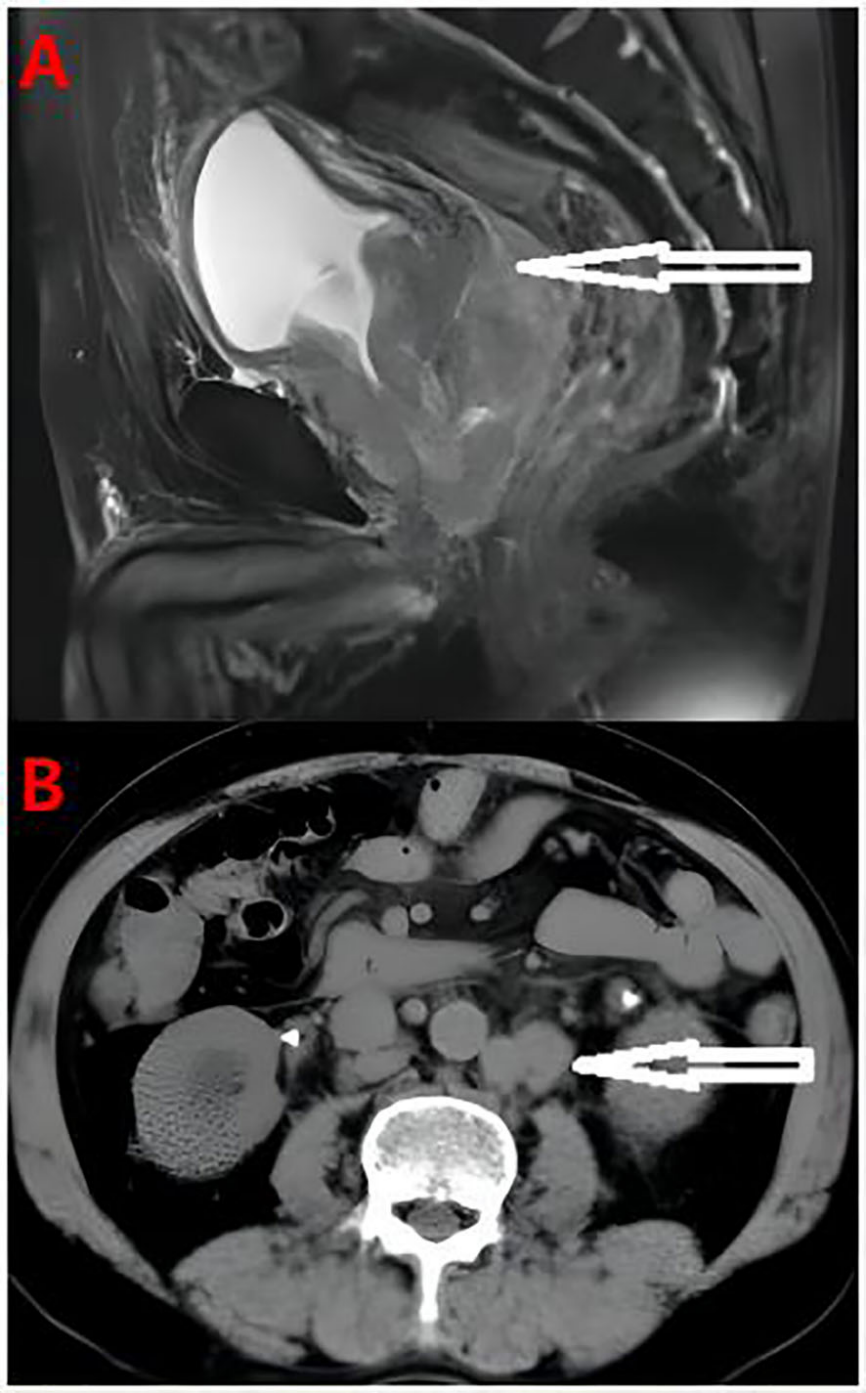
(A) Pelvic MRI Prostate cancer with bladder, bilateral seminal vesicle gland and peritoneum involvement, bilateral ureter and sigmoid colon involvement, secondary pelvic lymph node metastasis. (B) The abdominal computed tomography showed the tumor involved retroperitoneal lymph nodes.
